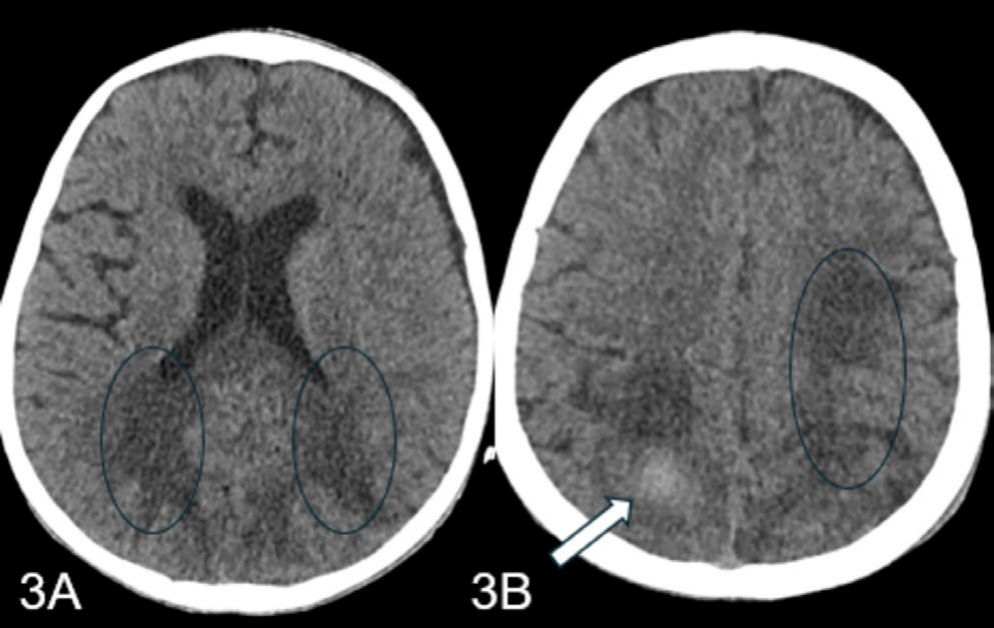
(A, B) Follow up CT scan shows gliosis in the posterior parietal and occipital lobes. Residual hemorrhage in the right posterior parietal lobe (3B arrow).
